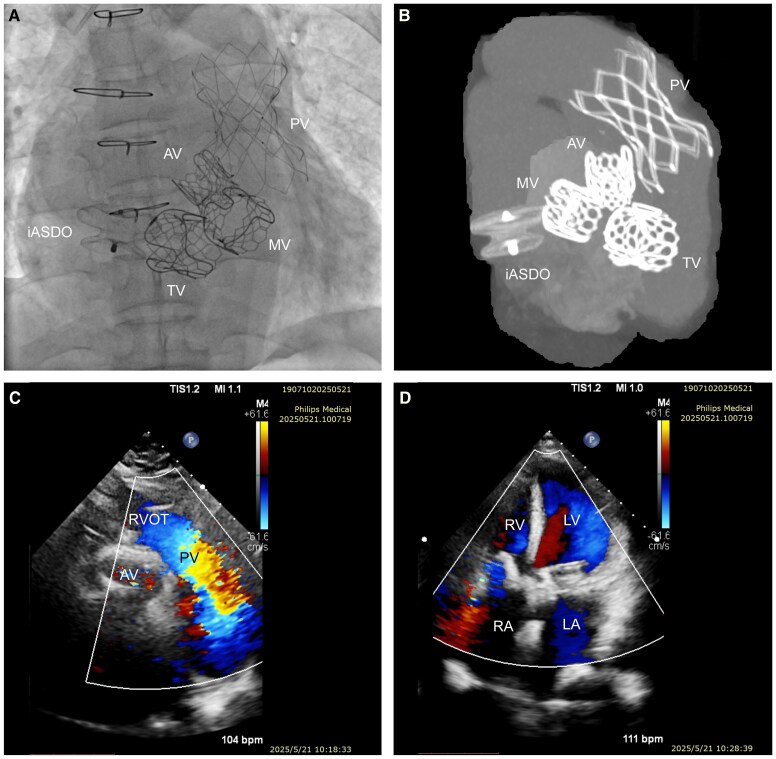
A 58-year-old woman with rheumatic heart disease and triple bioprosthetic valve replacement (aortic, mitral, tricuspid; 2011) presented with one year of worsening dyspnoea, chest tightness, and palpitations. Thirteen years after surgery, she exhibited NYHA III heart failure, irregular rhythm, and biphasic murmur. Echocardiography revealed advanced degeneration with severe stenosis and regurgitation of all prostheses, pulmonary valve regurgitation, right atrial thrombus (4.4 × 2.8 cm), biatrial enlargement, and biventricular dysfunction (LVEF 32%, RVEF 19%, FAC 26%). A fully percutaneous procedure was performed, sequentially deploying a balloon expandable PrizValve 23# in the aortic, PrizValve 26# in the mitral, PrizValve 29# in the tricuspid, and a self-expanding PT-Valve 40/26 in the pulmonary position. An iatrogenic atrial septal defect created during the procedure was closed to prevent paradoxical embolism (*Panels A* and *B*). Postoperatively, warfarin maintained the international normalized ratio between 2 and 3. At 15 months, all valves functioned well with thrombus resolution (*Panels C* and *D*). This is the first reported case of simultaneous transcatheter replacement of all four valves.
